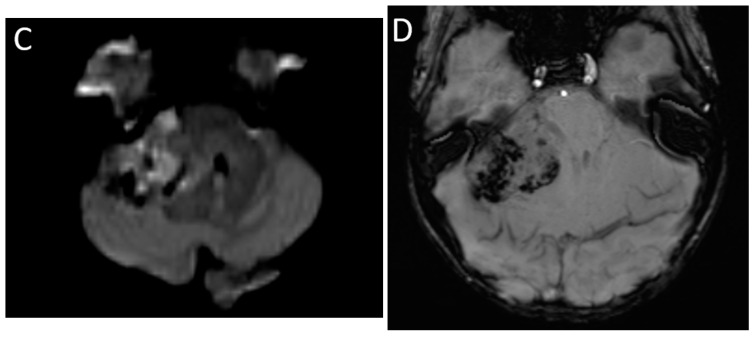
A 2.5-year-old girl with AT/RT. Axial T2 (A), post-contrast T1 (B), images demonstrate a heterogeneous lesion in the right cerebellopontine angle extending into the internal auditory canal. There is also a metastatic lesion in the left IAC (arrow). DWI (C) and SWI (D) imaging demonstrate restricted diffusion and susceptibility common in these lesions.
